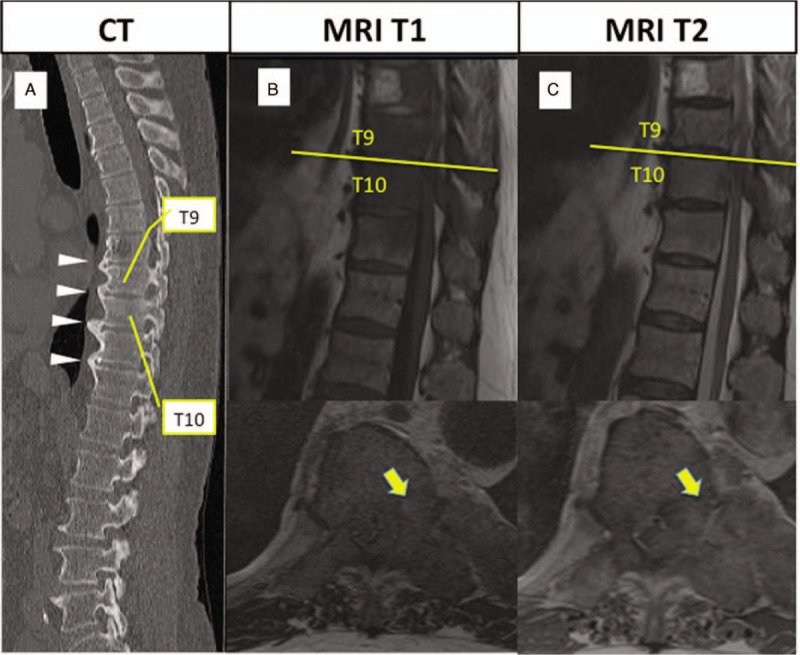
(A) CT scan showing fused vertebra from T8 to T12 because of ossification of anterior longitudinal ligament (white arrow). (B) T1-weighted and (C) T2-weighted magnetic resonance imaging scans showing spinal metastasis in the vertebra at T9 and T10 and left pedicle of T10 with rib metastasis (yellow arrow).
